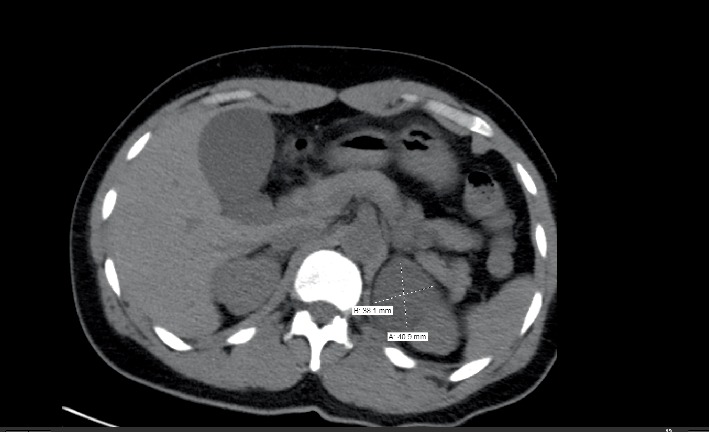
CT abdomen demonstrating adrenal tumor.
